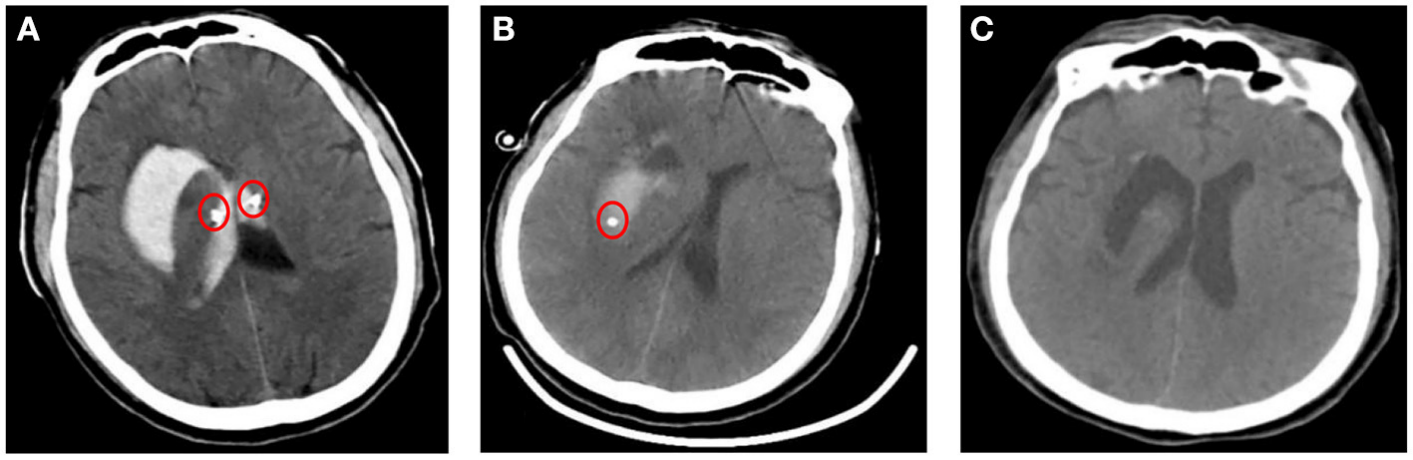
Imaging findings after treatments. (A, B) Images of a cranial CT after implantation of a drainage tube (red circle) though drilling in bilateral lateral ventricle and in the right basal ganglia. (C) CT after removing the drainage tube showed normal bilateral lateral ventricles and a cavity in right basal ganglia.
